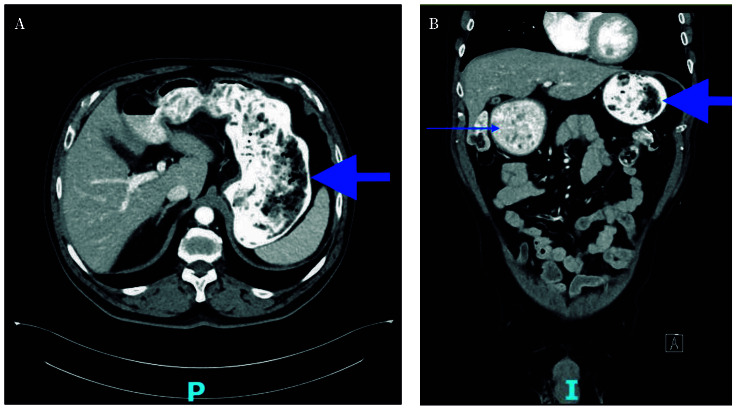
Computed tomography scan at admission. Axial (A) and coronal (B) view showing abundant, organized- appearing gastric content. Arrow: gastric chamber. Thin arrow: food retention at the pyloric antrum.
